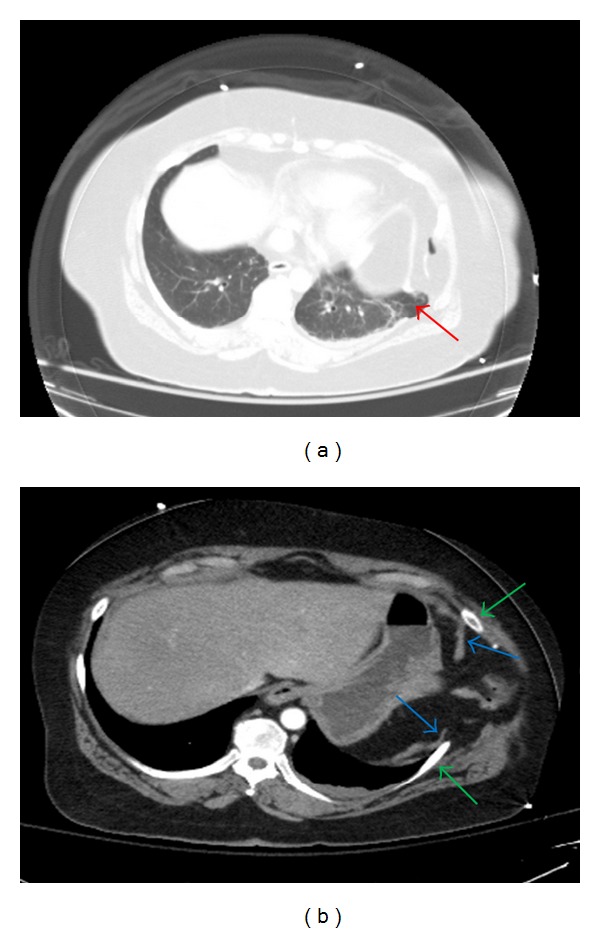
Axial CT images of the chest demonstrate herniation of lung beyond the thoracic cavity (red arrow) (a). There is discontinuity of the diaphragm (blue arrows) and widened intercostal space (green arrows). Herniation of abdominal contents is present including omentum and transverse colon into the chest and through the intercostal defect (b).
